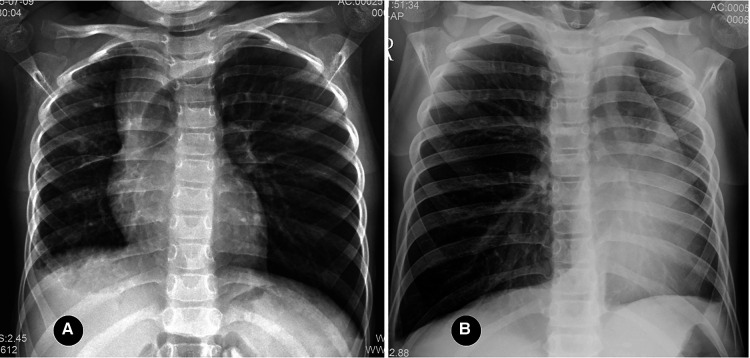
(A) right proximal interruption of the pulmonary artery (PIPA) and right-lung hypoplasia in a 4-year-old female patient. An anteroposterior radiograph of the chest shows a small volume of the right thoracic cage, the small volume of the right lung, the small hilum of the right lung, and slightly increased lucency of the right lung field. The left lung was hyperinflated, the mediastinum shifted to the right, and the diaphragm was slightly elevated on the right. (B) left-sided PIPA and left-lung hypoplasia in a 7-year-old male patient. An anteroposterior radiograph of the chest shows a reduced volume of the left thorax and lung, a mildly dense left lung field, and an increased volume of the right lung. The mediastinum is shifted to the left.
